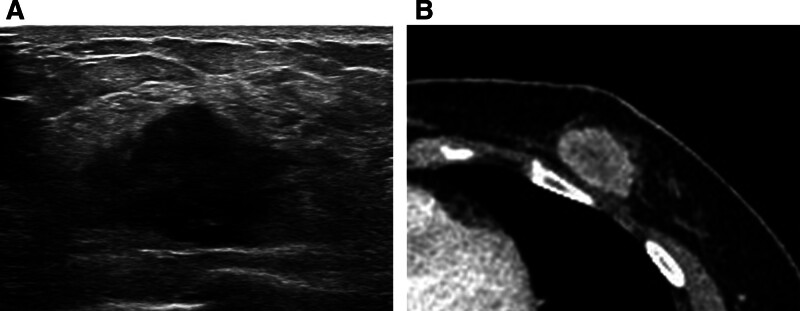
Breast ultrasound and CT findings of large cell neuroendocrine carcinoma. (A) The breast ultrasound image showed a hypoechoic mass with an irregular shape and microlobulated margins at the 6 o’clock position of the left breast, without posterior acoustic features or associated findings. (B) Chest CT revealed a rim-enhancing mass with a microlobulated margin in the left breast. CT = computed tomography.
